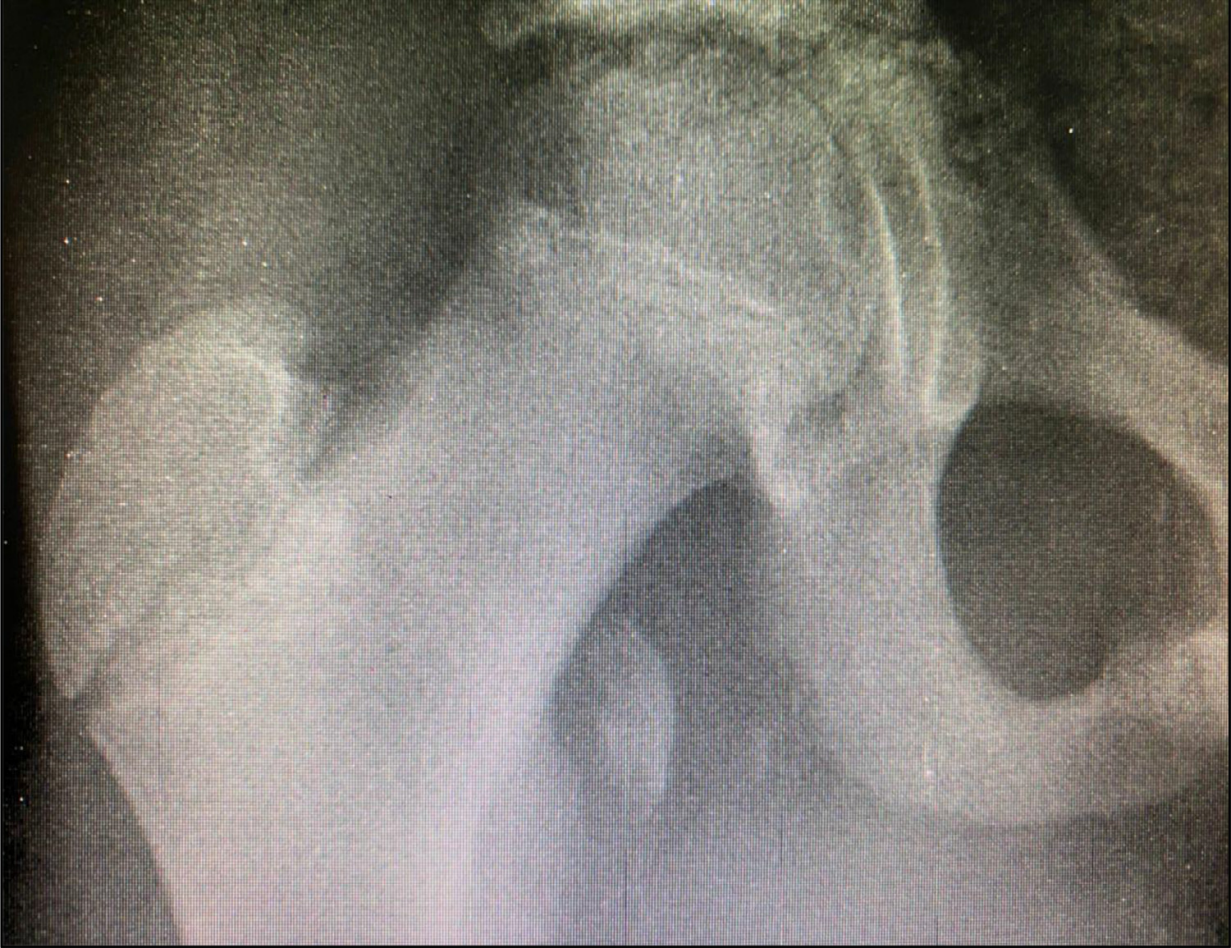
Radiograph of the right hip of a 12-year-old patient showing an avulsion of the right lesser trochanter 15 weeks after conservative management of similar injury to the left lesser trochanter.
